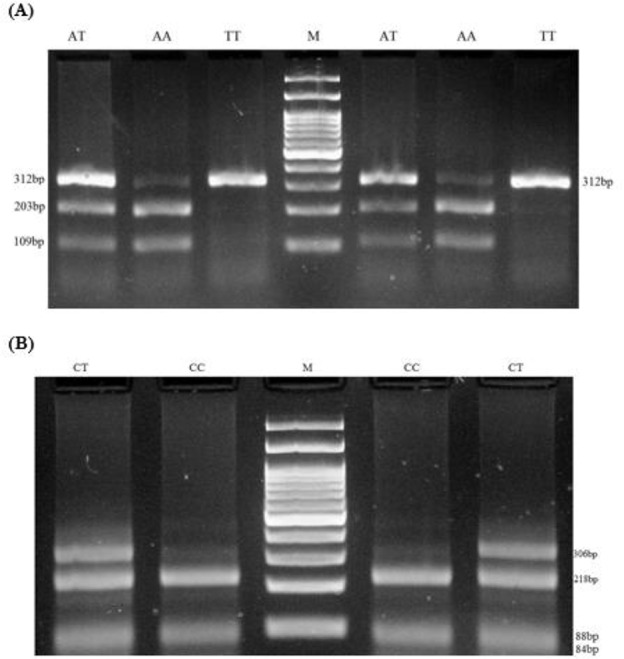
Electrophoresis pattern for detection of SNP in CAT rs7943316 A/T and GPX-1 rs1050450 C/T (A) RFLP -PCR products of CAT rs7943316 A/T, M: DNA marker (100 bp), product size (109, 203, 312bp), demonstrate genotype AT; product size(109, 203bp), demonstrate genotype AA and 312bp demonstrate genotype TT. (B) RFLP -PCR products of GPX-1 rs1050450 C/T, M: DNA marker (100 bp), product size (84/88, 218, 306bp), demonstrate genotype CT; product size(84/88, 218bp), demonstrate genotype CC
